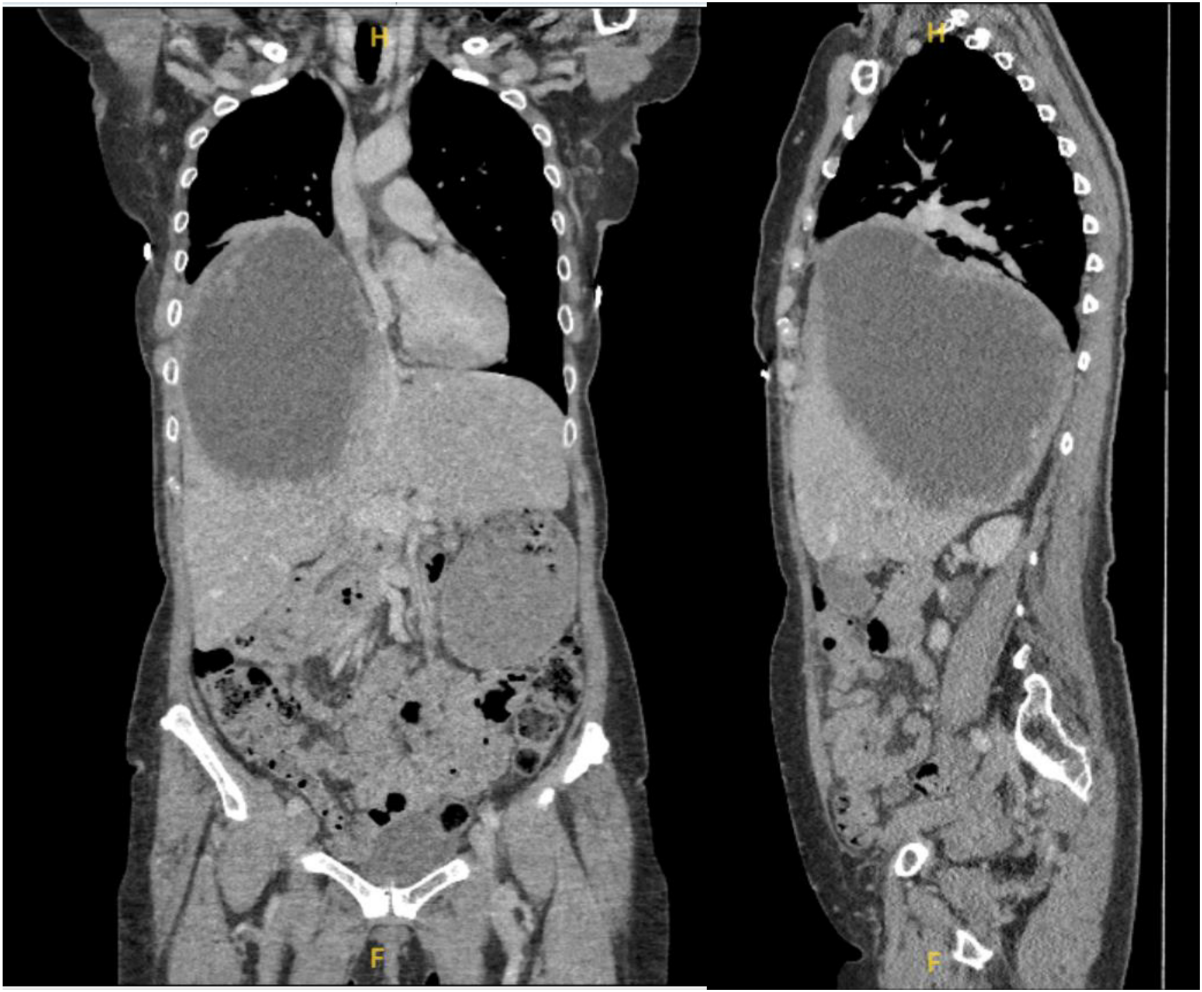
Preoperative imaging of the tumor. Sagittal and coronal CT scans demonstrating the extent of the hepatic tumor.
